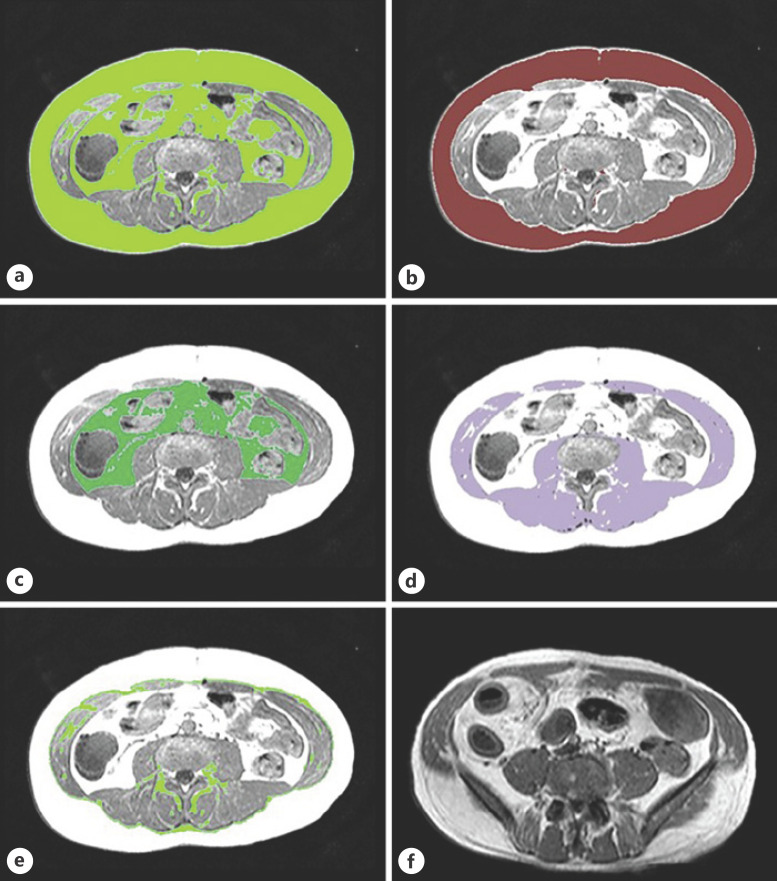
Segmentation of body composition based on cross-sectional area (CSA) measurements at the L3 inferior endplate level in MRE (a−e). a Total adipose tissue (TAT). b Subcutaneous adipose tissue (SAT). c Visceral adipose tissue (VAT). d Skeletal muscle tissue (SMT). e Intramuscular adipose tissue (IMAT). f Creeping fat (CrF), T1-weighted MRE image shows fatty deposition along the mesenteric border of inflamed bowel segment.
